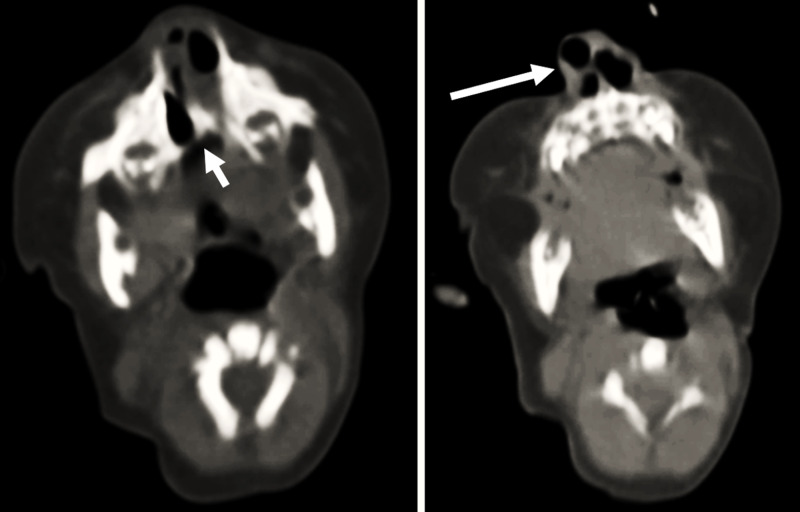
Cranial CT Cranial CT images demonstrating the bilateral choanal atresia (short arrow) and the right ectopic nostril (long arrow).
